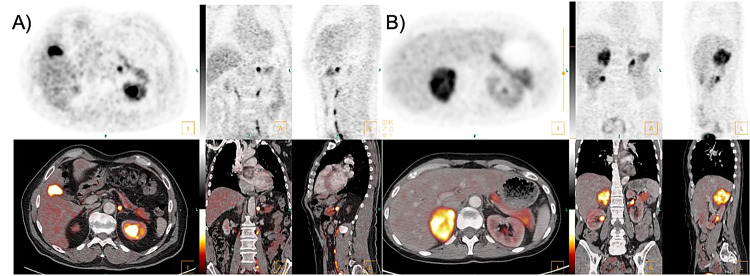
Patient examples with adrenergic and noradrenergic phenotype. (A): [^18^F]FDOPA PET/CT of a 65-year-old male patient with a small left-sided pheochromocytoma with strong radioligand uptake. The adrenal mass was detected incidentally on a CT scan performed due to suspected urolithiasis. The patient had a two-year history of intermittent vertigo and tremor. The levels of metanephrine (67 ng/l) and 3-methoxytyramine ( < 10 ng/l) were within the normal range, the normetanephrine concentration was slightly elevated at 206 ng/l (norm < 200 ng/l). [^18^F]FDOPA PET/CT showed increased [^18^F]FDOPA uptake (SUVmax 14.7, SUVmean 7.1, MTV 2.3 ml, TLU 16.4 ml*SUV). The patient declined genetic testing. (B): [^18^F]FDOPA PET/CT of a 53-year-old female patient with right-sided pheochromocytoma with a typical rim-enhancement and central cystic parts. The patient presented for the differential diagnosis of an adrenal incidentaloma. Clinically, the patient presented with palpitations and an arterial hypertension. While the metanephrine concentration of 60 ng/l was within the normal range, a significantly elevated normetanephrine concentration of 3190 ng/l (norm < 200 ng/l) was found. 3-methoxytyramine was moderately elevated at 43.0 ng/l. Significantly elevated levels of chromogranin A (844 µg/l) were detected. On [^18^F]FDOPA-PET/CT, the mass showed increased radioligand uptake (SUVmax 9.6, SUVmean 5.8, MTV 61.1 ml, TLU 354.4 ml*SUV). Genetic analysis showed no evidence of a hereditary origin
